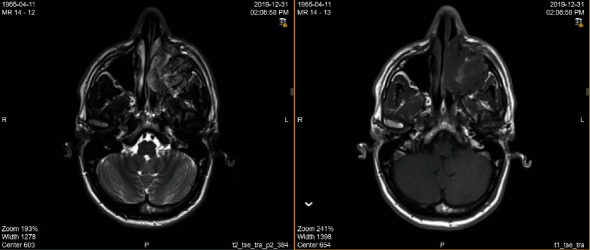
Orbital MRI images show a large mass occupying and destructing the left maxillary sinus with extension into the left nasal cavity and the adjacent retromaxillary space.
